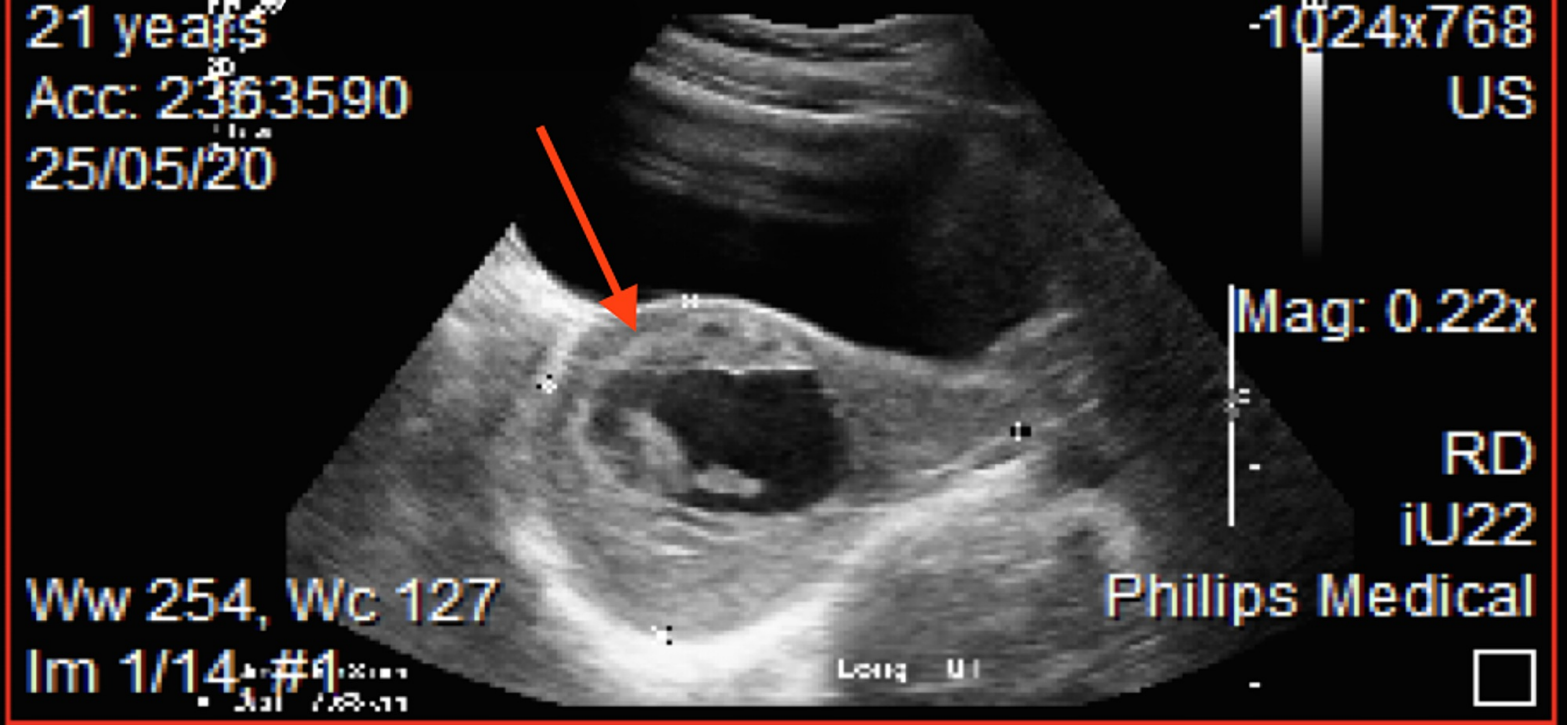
Retained tissue with positive Doppler
